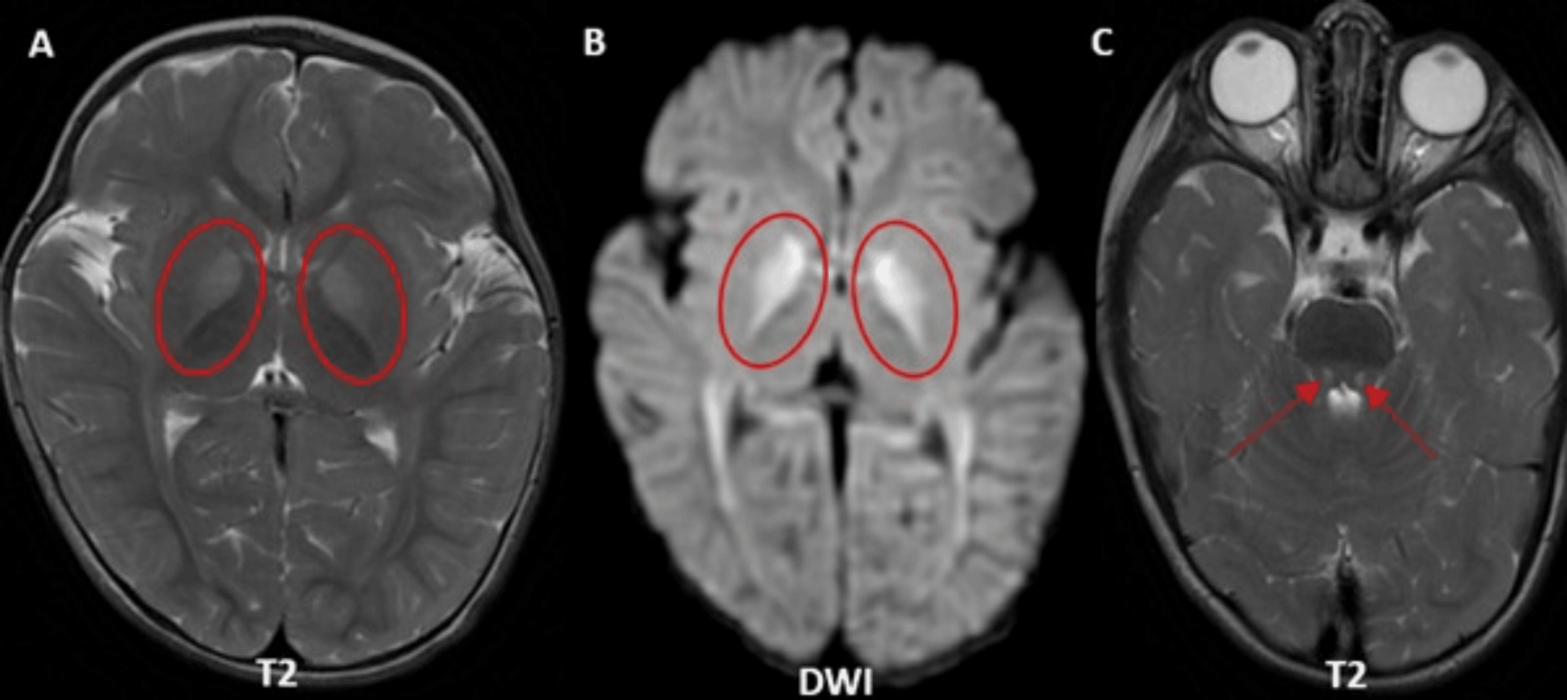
Axial sections of MRI brain (globus pallidi and central tegmental tracts). (A) Bilateral symmetrical T2 hyperintensities in the globus pallidi (red circles). (B) Corresponding restricted diffusion on DWI in the globus pallidi (red circles). (C) Bilateral symmetrical T2 hyperintensities in central tegmental tracts (red arrows). MRI: magnetic resonance imaging; DWI: diffusion-weighted imaging
